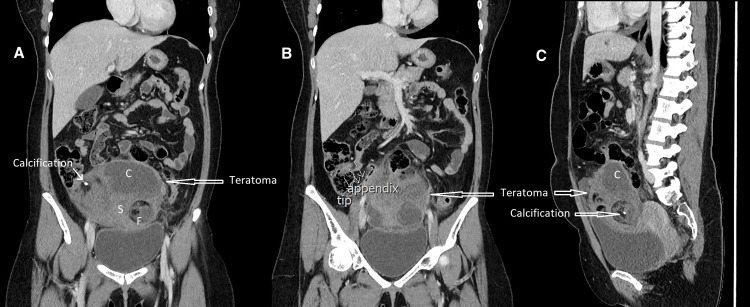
CT scan images of the abdomen and pelvis, with intravenous contrast, in the portal-venous phase A: Coronal view with large teratoma with calcification, solid (S) and cystic (C) areas and fatty tissue (F). B: Coronal view of the normal-looking appendix. Teratoma can be seen right next to the appendix. C: Sagittal view of the teratoma with cystic (C) and solid tissue, fatty tissue and calcification.
